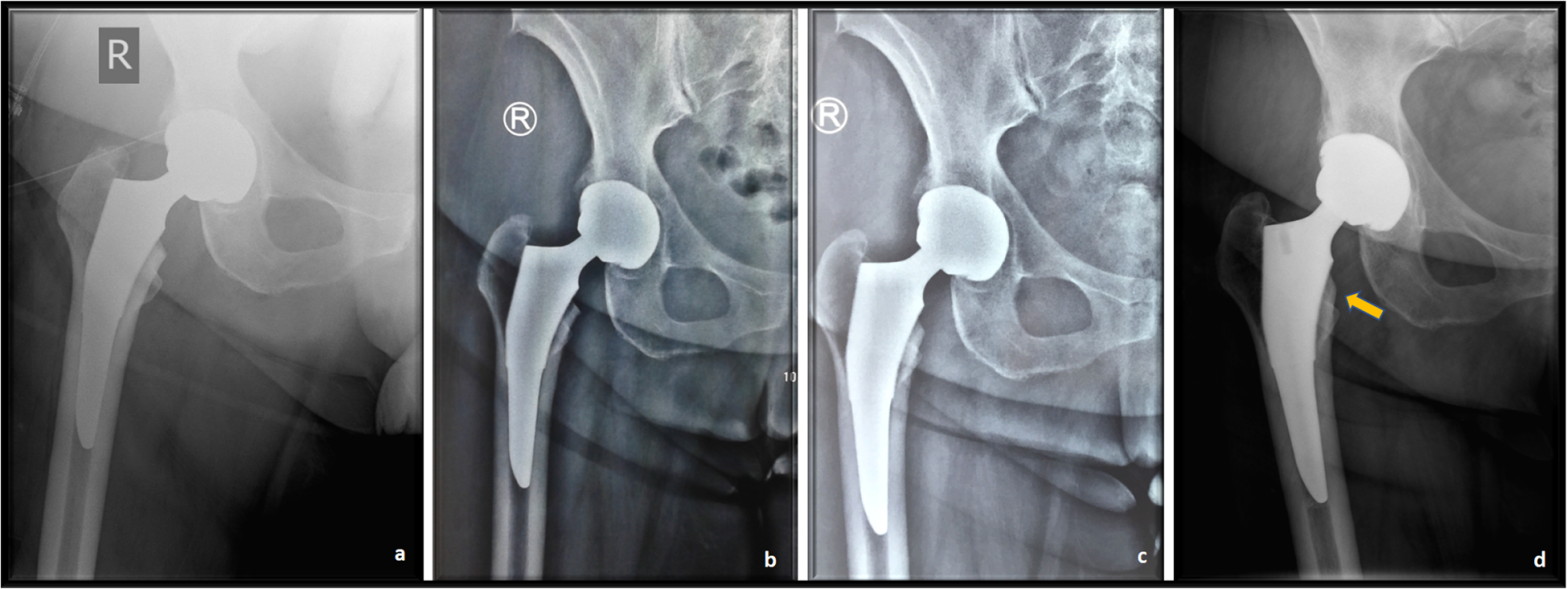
Anteroposterior pelvis radiographs of a 59-year-old woman who underwent a right total hip arthroplasty with the Tri-Lock BPS. a First day, b 6 months, c 1-year, and d 2 years postoperatively. Grade 1 stress shielding effect was noted 2 years after implantation
